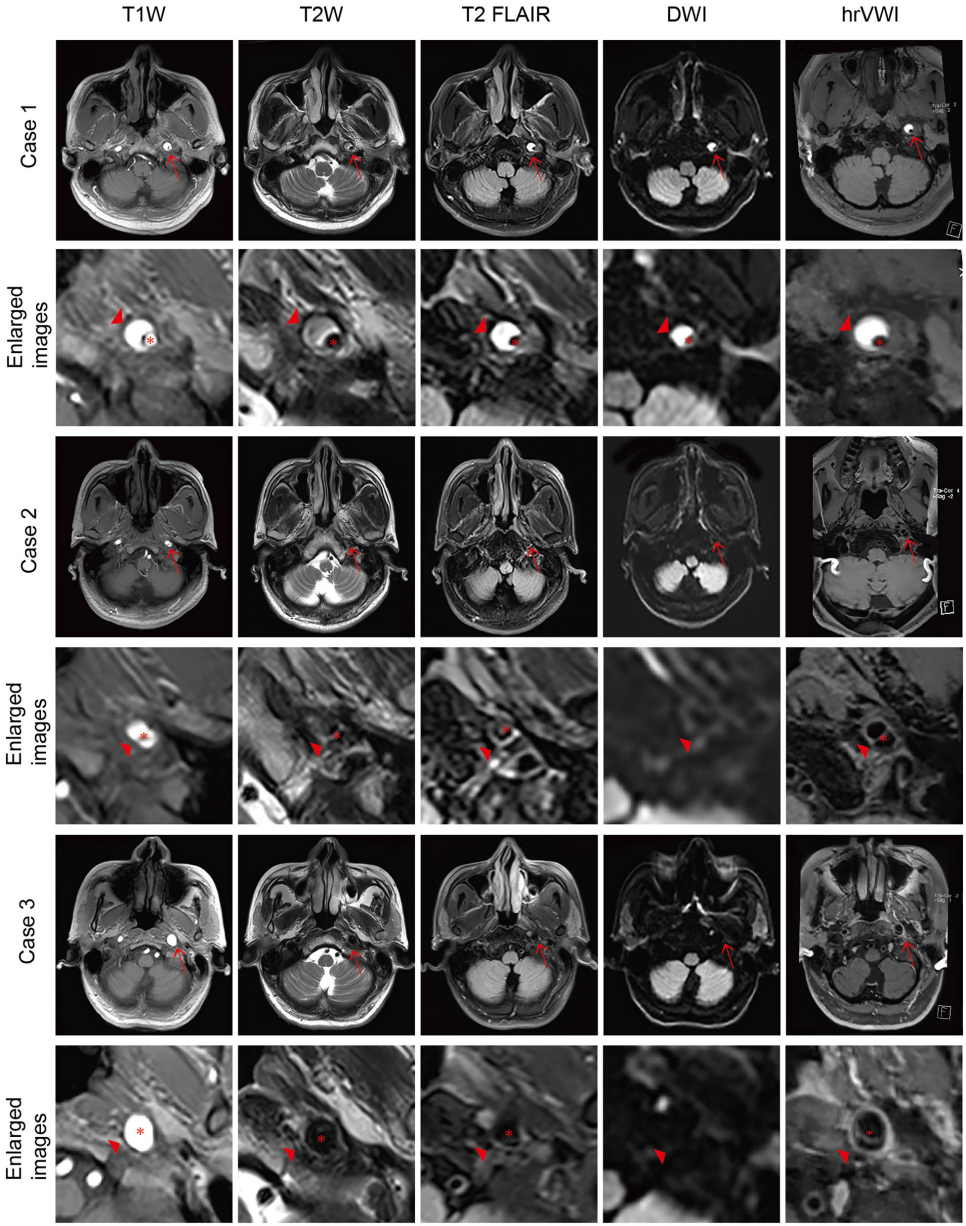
Representative brain MRI and high-resolution vessel wall magnetic resonance imaging (hrVWI) of extracranial internal carotid artery (EICA) dissection with typical signs. (Case 1) The dissection in the distal third of the left EICA with a mural hematoma on axial brain MRI and hrVWI sequences (arrow), showing crescentic hypersignals (arrowhead) with a tiny lumen. (Case 2) A left EICA dissection with an intimal tear/double lumen on axial brain MRI and hrVWI sequences (arrow), showing an isoisointense line crossing the dilated lumen (arrowhead). The lesion is ambiguous on DWI and distinct in other sequences. (Case 3) A left ICA dissection with a dissecting aneurysm on axial brain MRI and hrVWI sequences (arrow). The lesion is negative on DWI and shows vessel dilation on other sequences (arrowhead). The intimal flap is only seen on the hrVWI. The red asterisk indicates the lumen.
